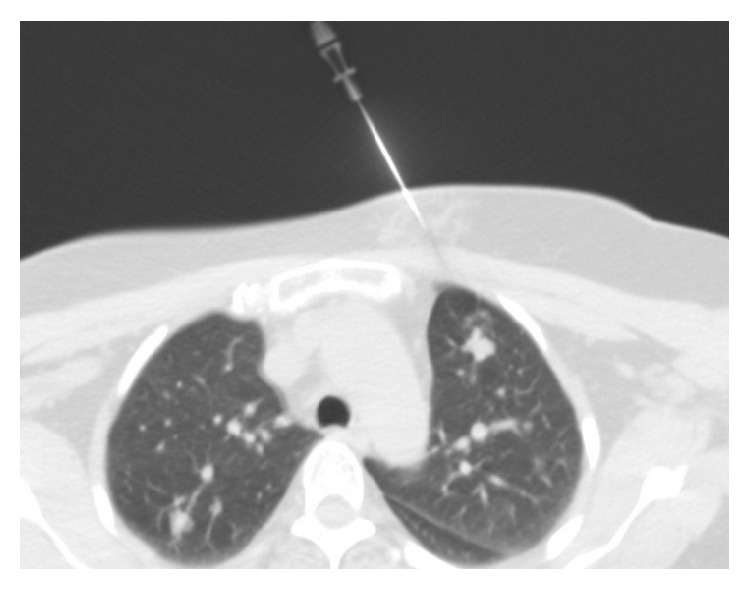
The needle advances toward the nodule in the anterior segment of the left lung during transthoracic biopsy.
